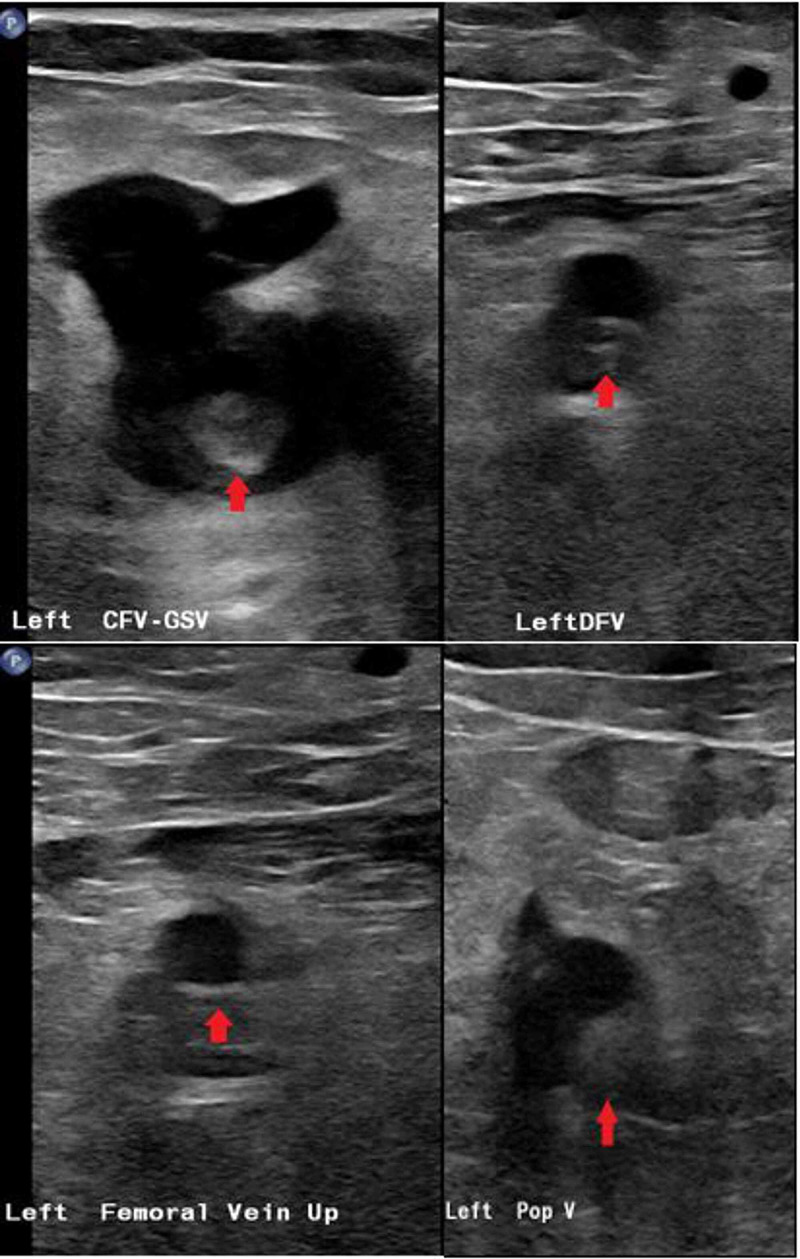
Ultrasound of the left lower extremity vein Extensive lower extremity deep venous thrombosis of the left lower extremity vein (red arrows). CFV, common femoral vein; GSV, Great saphenous vein; DFV, Deep femoral vein; Up, Upper area; Pop V, Popliteal vein
